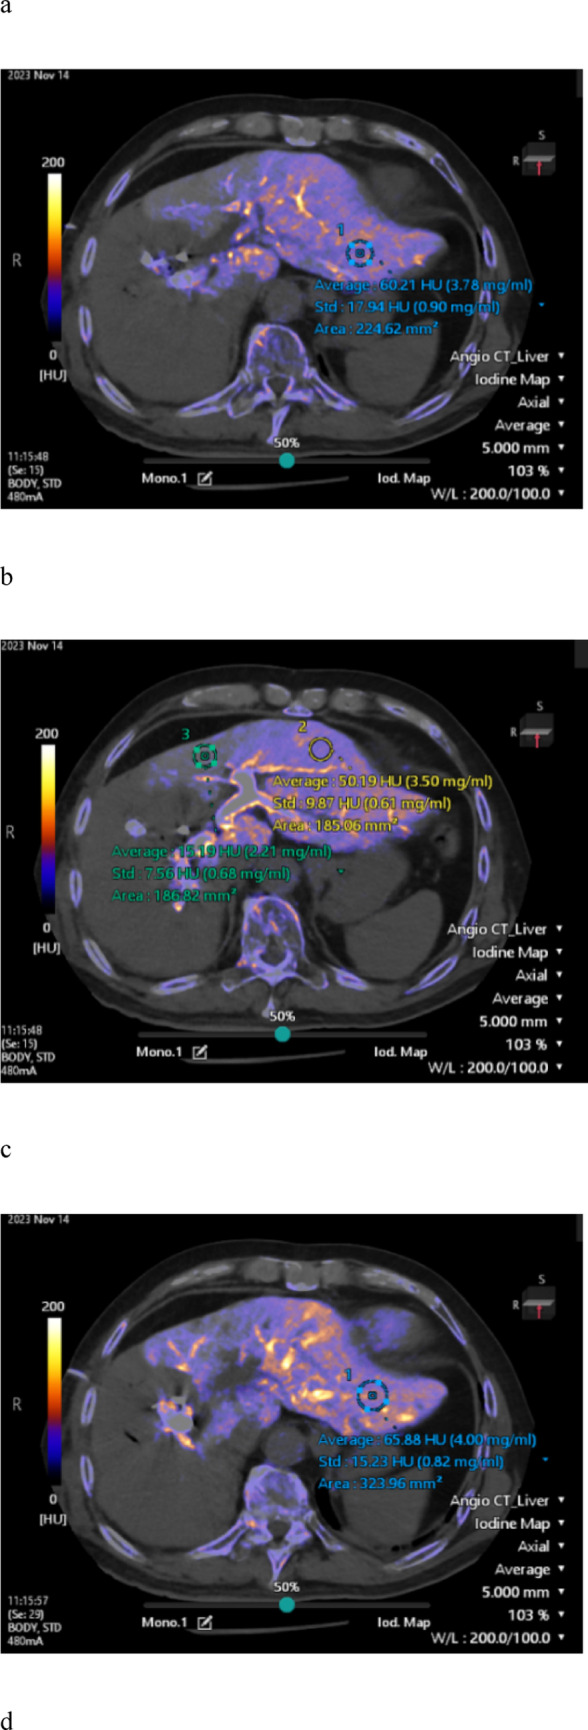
A 72-year-old male patient with perihilar cholangiocarcinoma underwent DECT, from which iodine maps were generated. Iodine concentrations were measured in both the early and late phases using ROIs larger than 1 cm^2^, placed in each segment of the left hepatic lobe (S2, S3, and S4). In the iodine map images, a shows a representative ROI placed in segment S2 during the early phase, whereas b shows representative ROIs placed in segments S3 and S4 during the early phase. Similarly, c shows a representative ROI in segment S2 during the late phase, and d shows representative ROIs in segments S3 and S4 during the late phase. DECT, dual-energy computed tomography; ROI, region of interest
